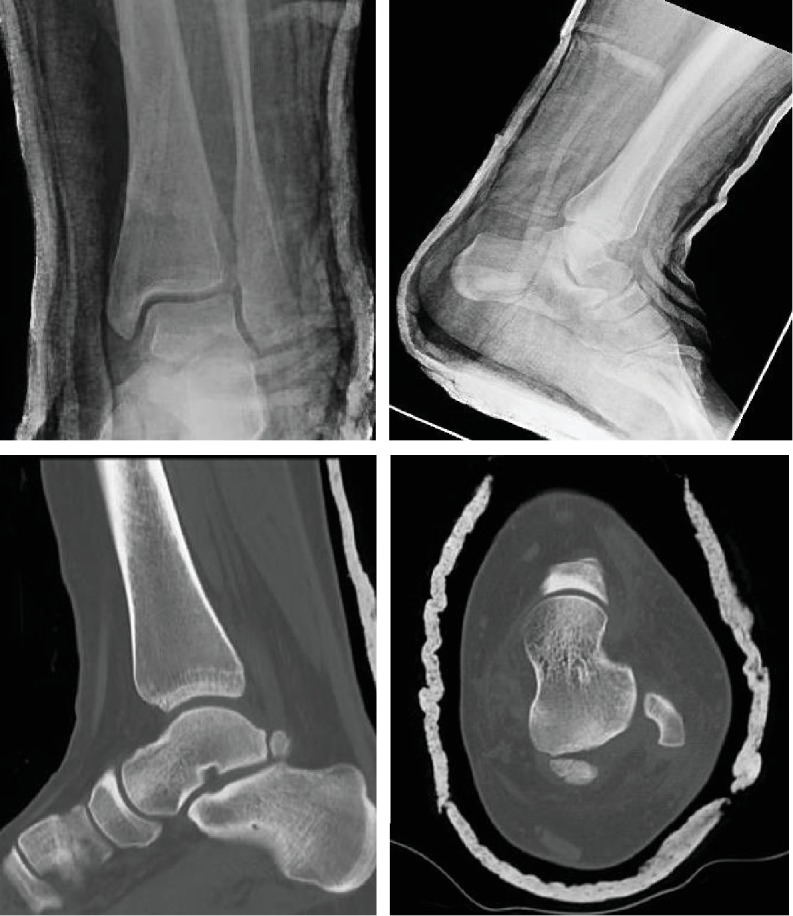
Mortise and lateral radiographs demonstrating reduced tibiotalar joint. Axial and lateral CT scans demonstrate displaced os trigonum without the fracture.
